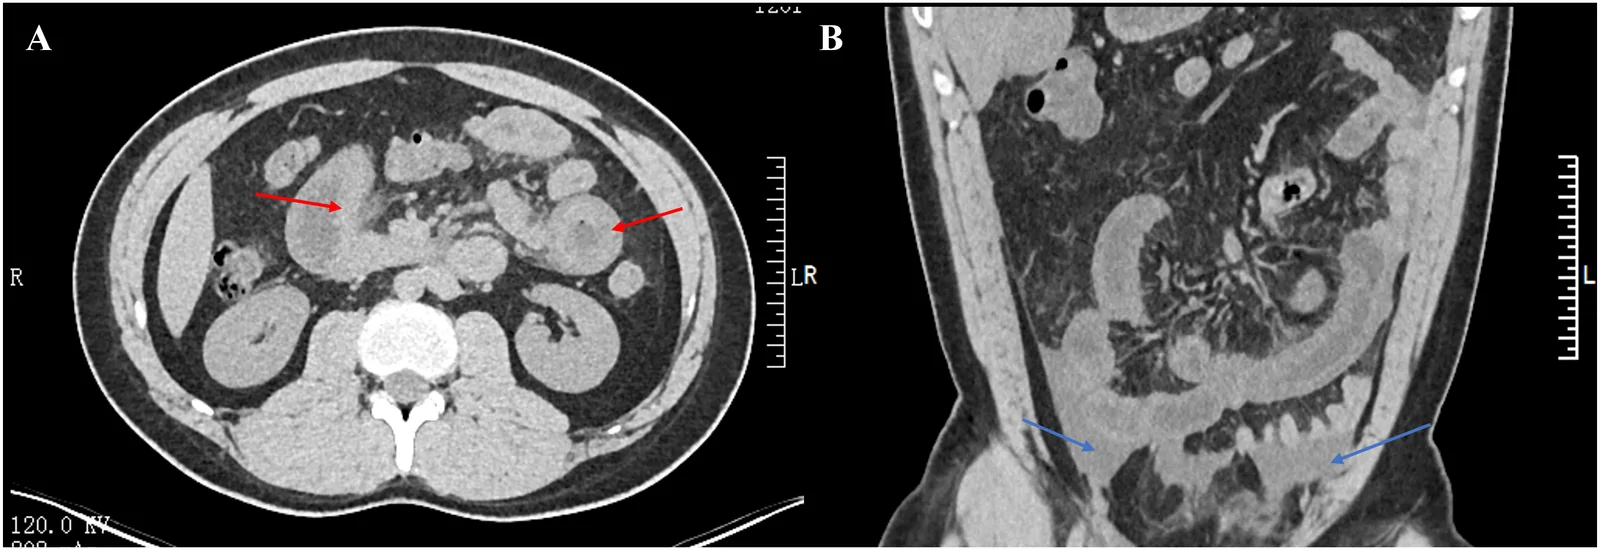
Computed tomography showing circumferential thickening of the duodenum and proximal jejunum (red arrows) with moderate ascites (blue arrows).
